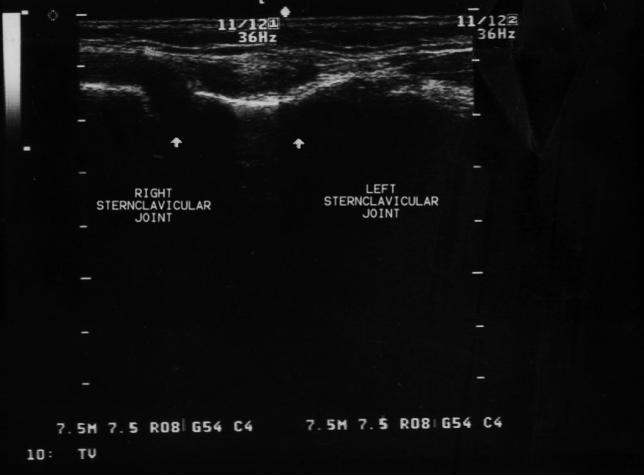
Ultrasonography of the Sternoclavicular Joints The image from an ultrasonography examination shows a normal left sternoclavicular joint, the clavicle appearing in continuity with the sternum. In the right sternoclavicular joint, the continuity is lost, reflecting the joint distention.
